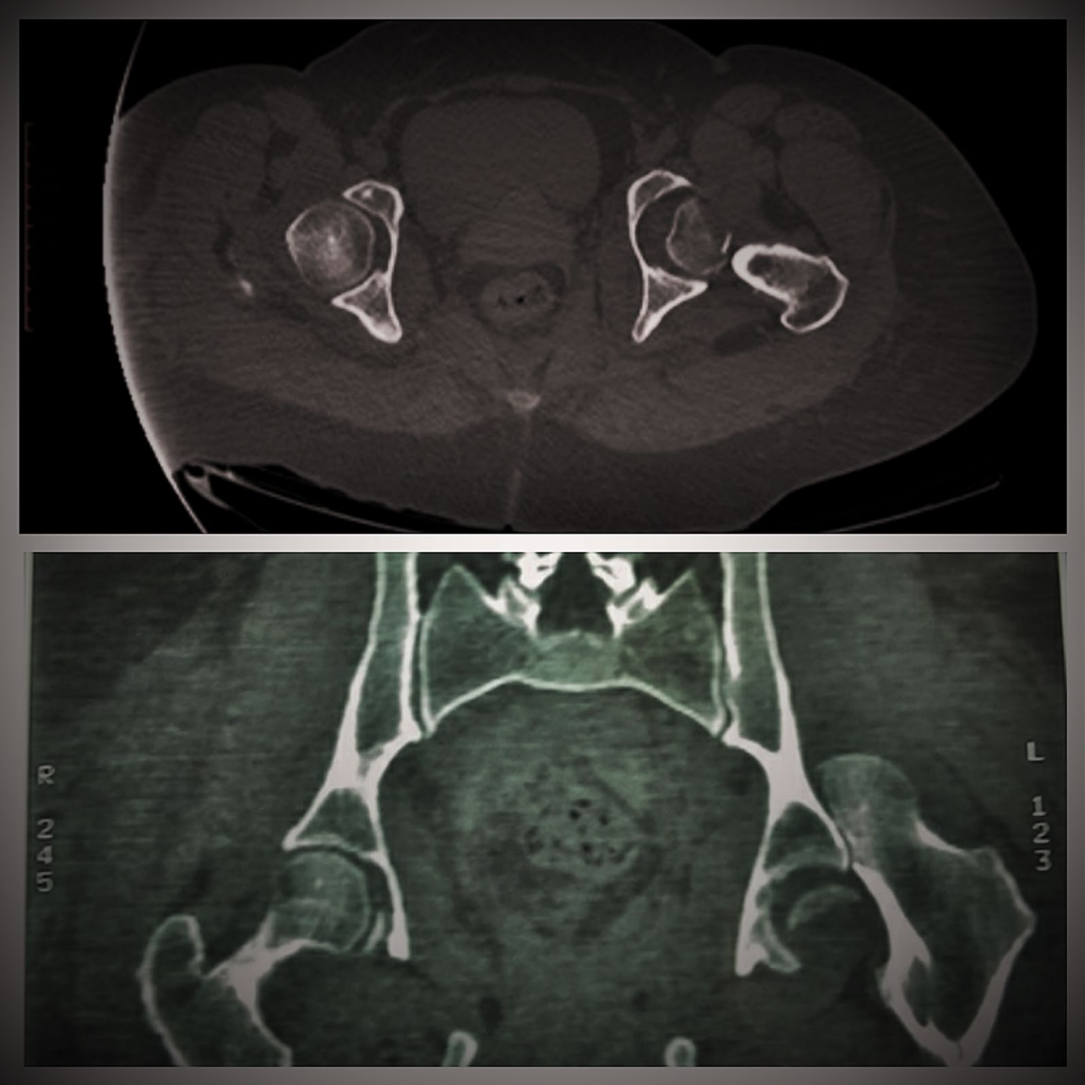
A Preoperative CT scan showing head femur fracture (axial view). B Preoperative CT scan showing head femur fracture (coronal view)
